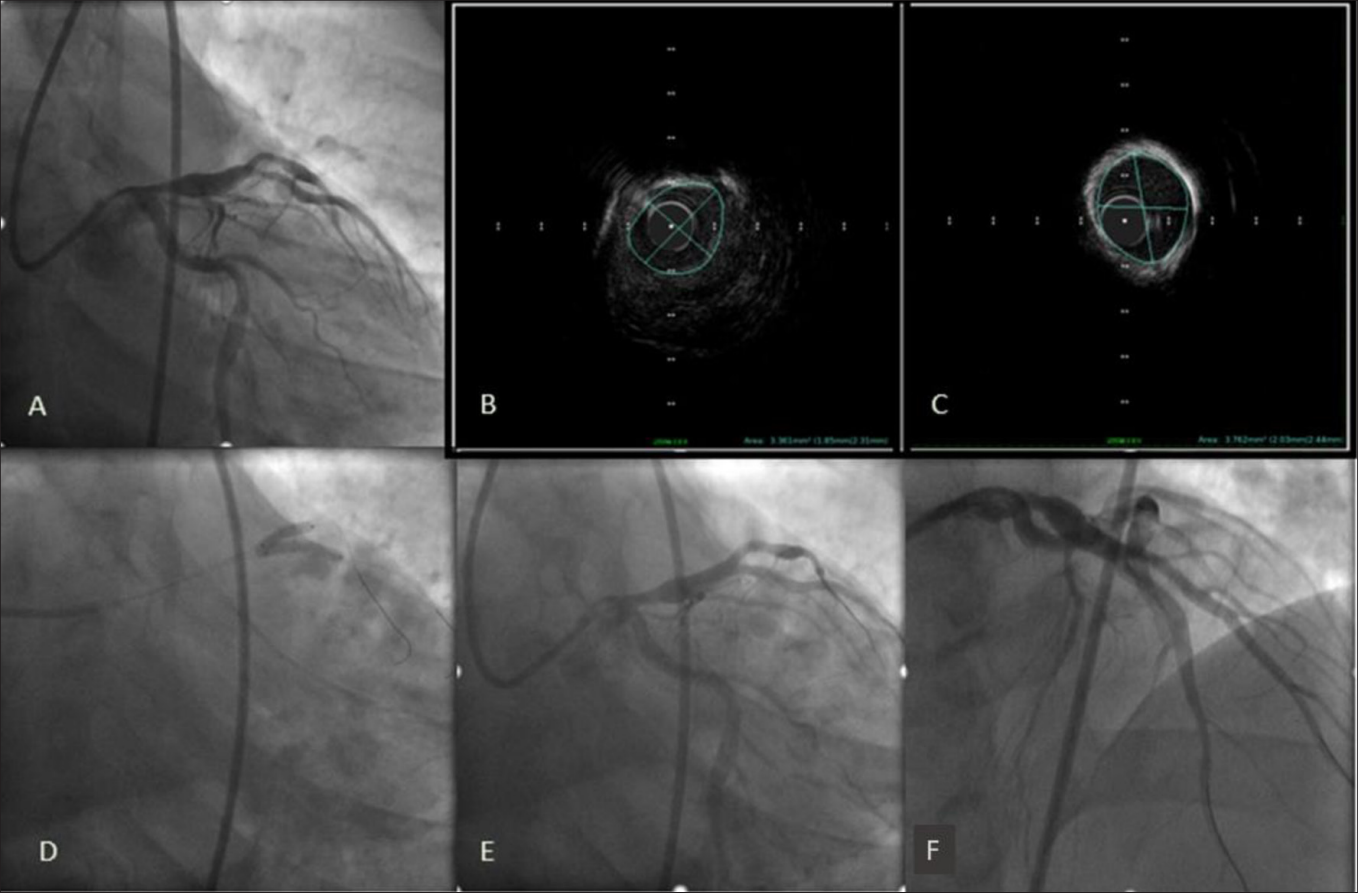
(A) Angiography 48 hours later showed near thrombus resolution. (B) Intravascular ultrasound of the mid-left anterior descending artery (C) and first diagonal branch (D1) showed minimal lumen area of 3.7 mm^2^ and 3.4 mm^2^, respectively. (D) Simultaneous kissing stent placement in the left anterior descending artery and the D1 branch. (E, F) Final angiography revealed thrombolysis in myocardial infarction-3 flow and no residual stenosis.
